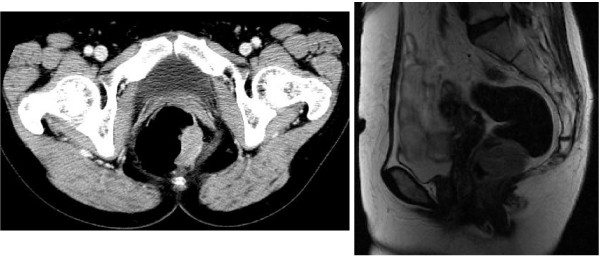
CT and MRI findings.
